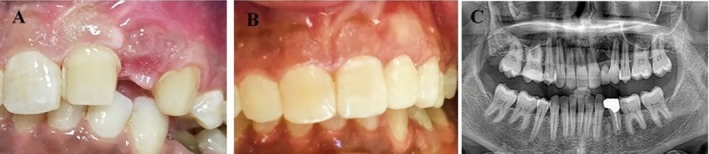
Clinical photographs after 5‐month (A) and 5‐year follow up (B). OPG radiograph after 5‐year follow‐up (C).
